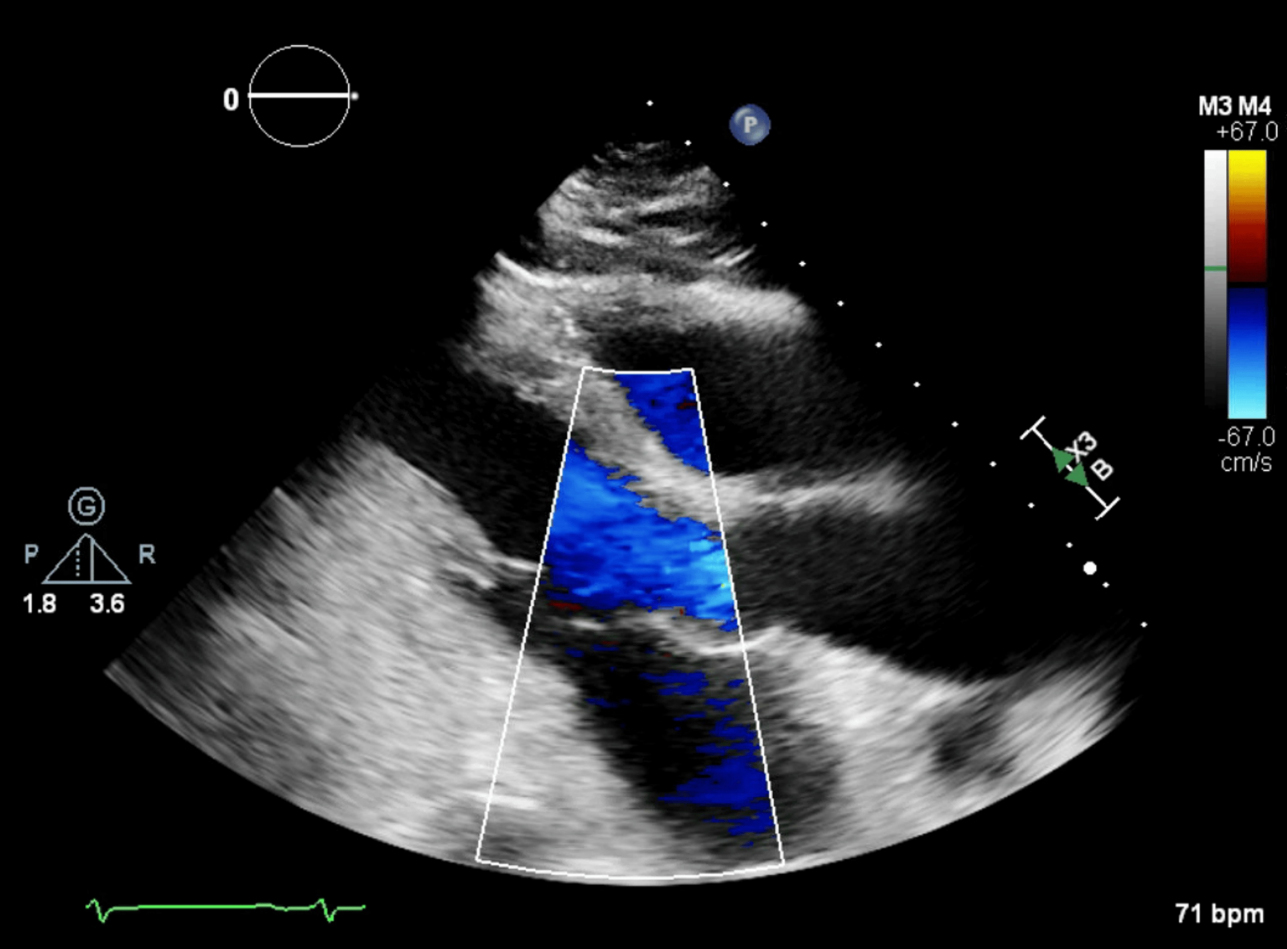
Echocardiography with Doppler flow demonstrating air in the left atrium
